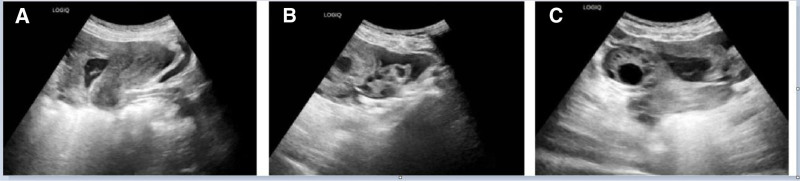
Transabdominal ultrasound characteristics for the first-time examination. (A) Transabdominal ultrasound showed endometrial thickness of 1.1 cm and pelvic effusion depth of 3.0 cm. (B) The image of the left ovary. (C) The image of the right ovary.
